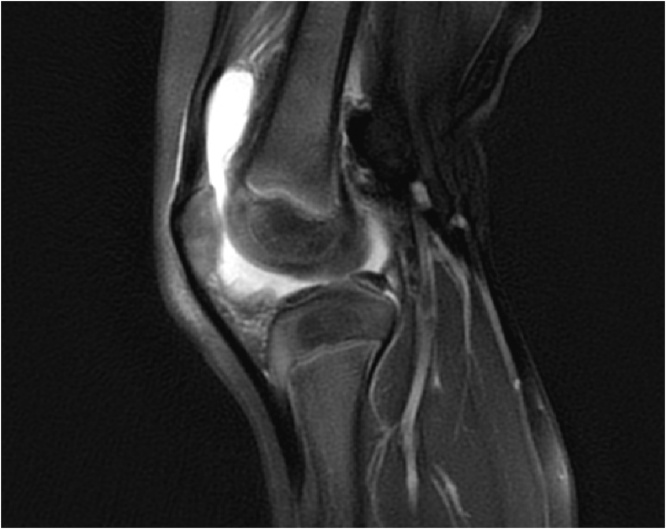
MRI right knee (Sagittal T2 Fat Suppression sequence): Large Knee Effusion with no abnormal bone marrow signal or fluid collection to suggest abscess.
